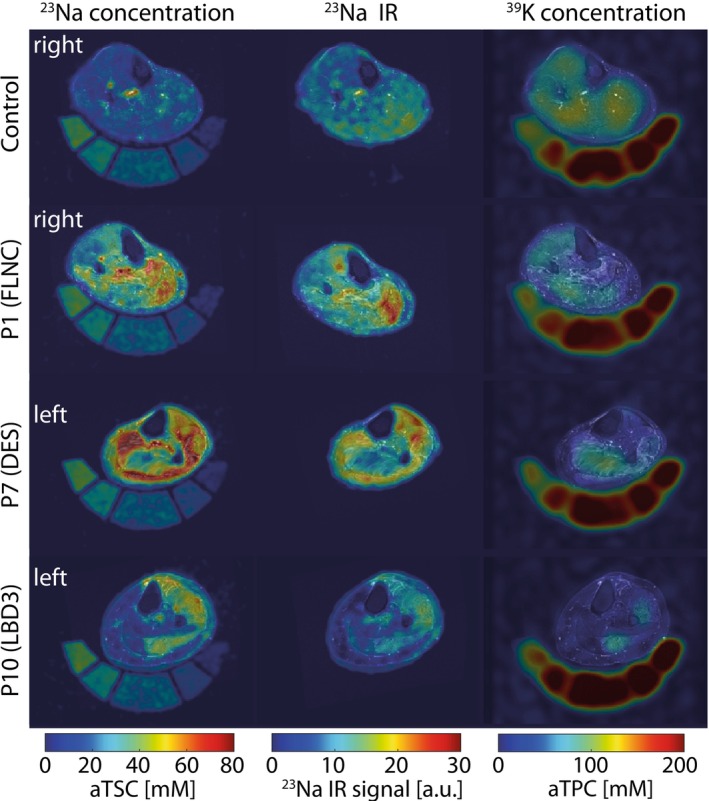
Maps of lower legs of myofibrillar patients (MFM) demonstrating different degrees of increase of apparent tissue sodium concentration (aTSC) and ^23^Na IR signal in non‐complete fatty replaced muscles and decrease of apparent tissue potassium concentration (aTPC) in all muscular regions. Note the increased aTSC/^23^Na IR signal and reduced aTPC in various muscle regions of three myofibrillar myopathy as well as the markedly reduced aTSC and ^23^Na IR signals in the medial part of the gastrocnemius and the soleus muscles of P10, which displayed a high degree of fatty replacement in T_1_ weighted images. ^23^Na and ^39^K MR images are overlaid to corresponding ^1^H T_2_‐weighted STIR images. The same healthy control subject and myofibrillar myopathy patients as in Figure 1 are shown.
